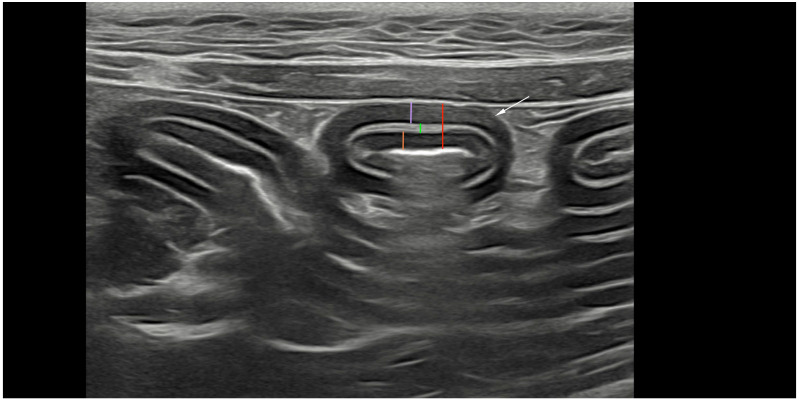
Ultrasonographic transversal image of the jejunum (white arrow; 8–12 MHz linear transducer). The red marking indicates the total wall thickness, the purple marking the lamina muscularis, the green marking the submucosa, and the orange marking the mucosa.
